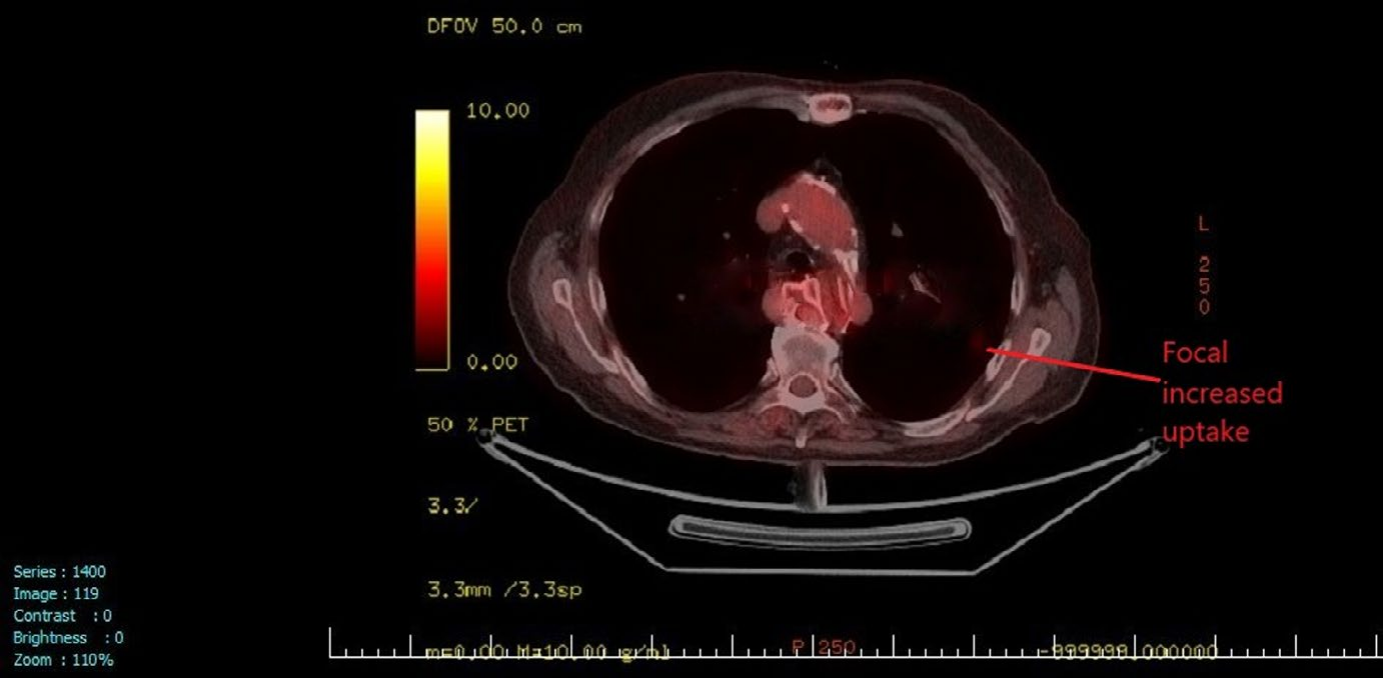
PET/CT scan revealing a 1.1 cm nodule at the upper right lung field (SUV_MAX_:5,9)
